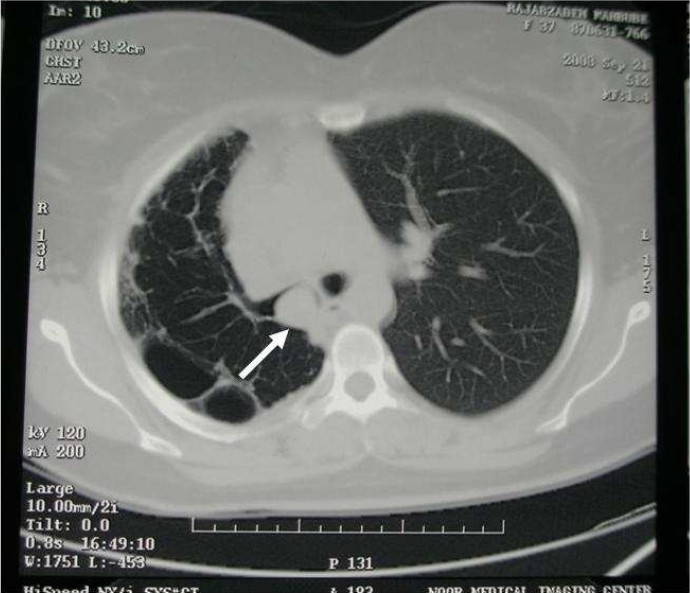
The tumor in proximal right main bronchus (RMB) in chest CT scan
